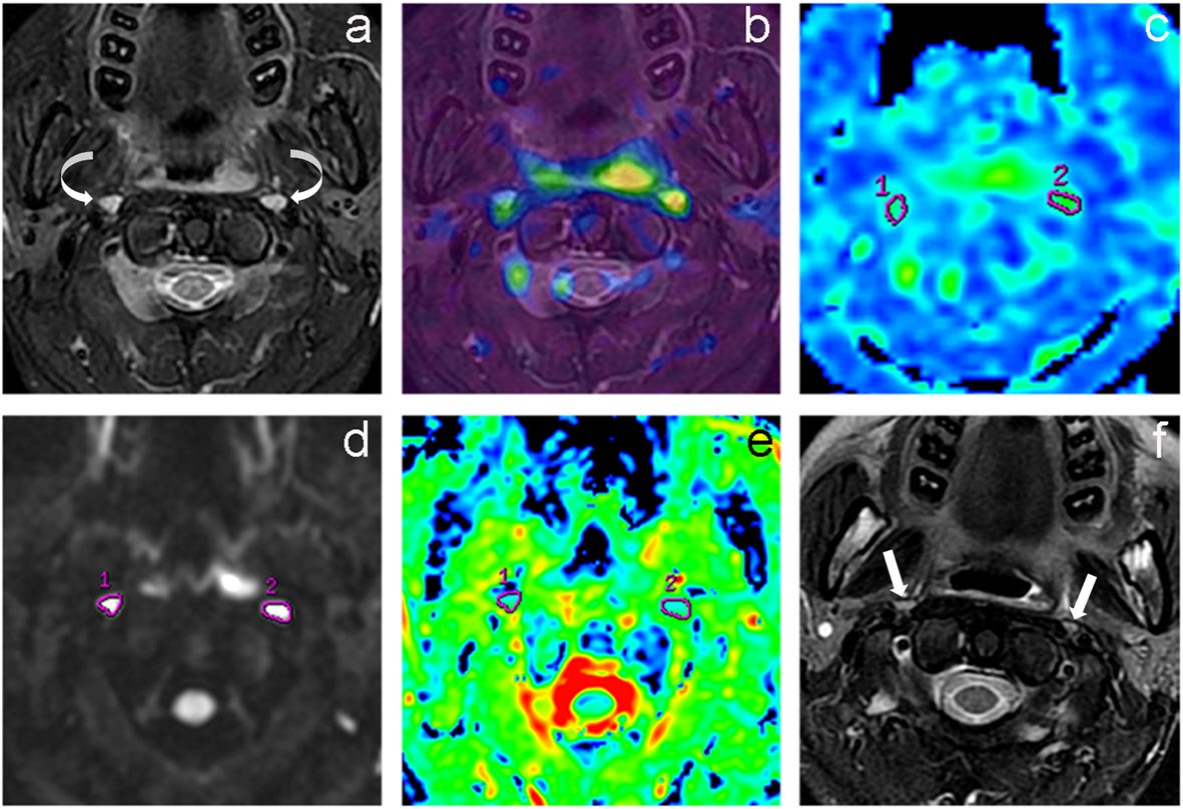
F29, NPC with bilateral metastatic RLNs. Axial T2WI before treatment (a) showed an irregular mass with heterogeneous slightly high signal intensity in the left lateral portion of the nasopharyngeal cavity and bilateral RLNs (curved arrows) with the size as long axis × short axis of 7 × 5 mm (right) and 8 × 5 mm (left), respectively. The primary tumor and RLNs demonstrated higher perfusion compared with the surrounding tissue on the fused image obtained by ASL and T2WI (b), and the map of BF (c). ROI of the RLNs were delineated on the map of BF (c) and multi-b-value DWI with b value at 800 s/mm^2^
